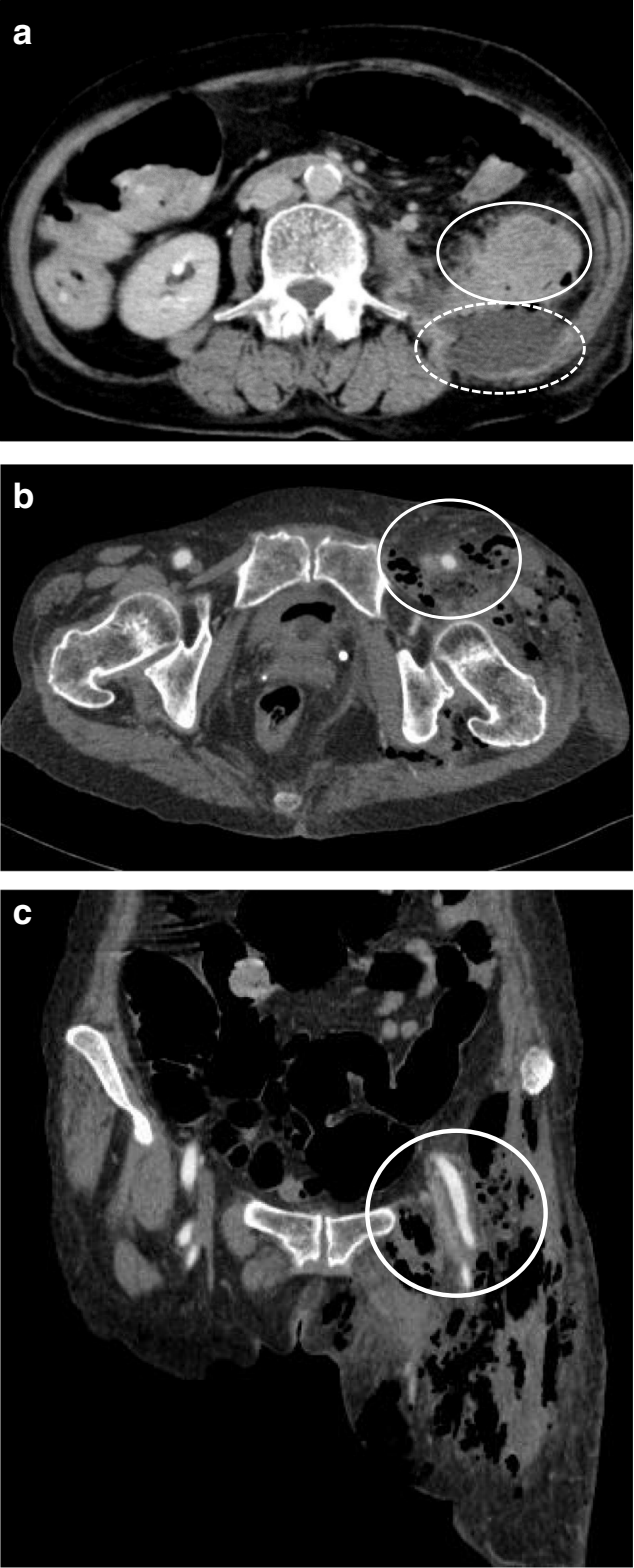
a Computed tomography showed a thickened wall of the descending colon (circle) and abscess formation around this area (dotted circle). b, c Emphysema was present from the retroperitoneal abscess around the descending colon to the left thigh through the left femoral region (circles)
